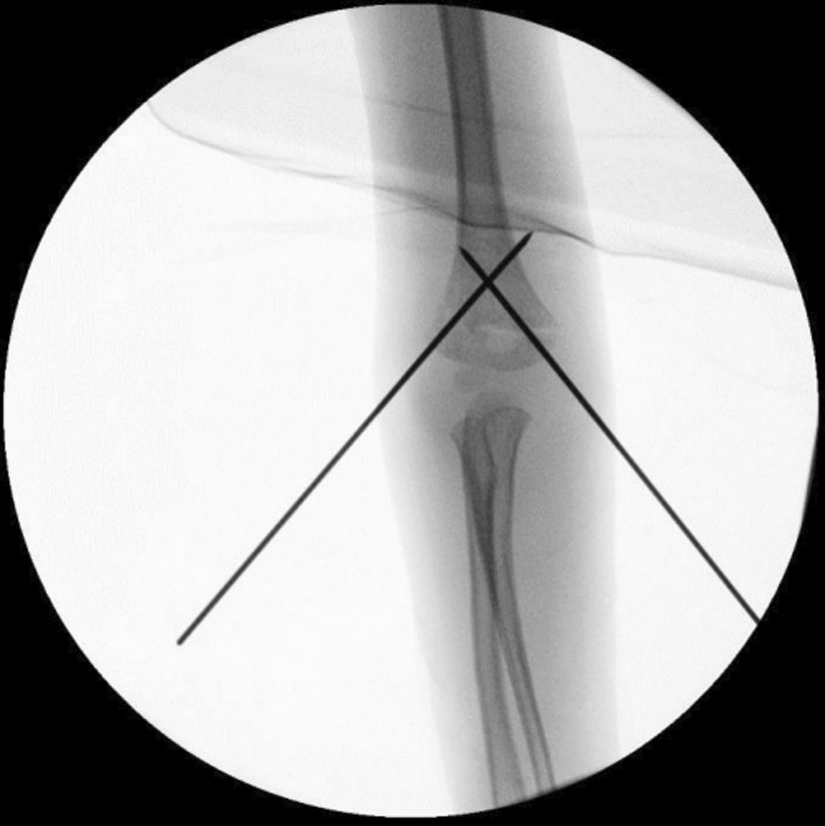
Intraoperative antero-posterior radiograph: reduction and pinning fixation with two crossed pins
